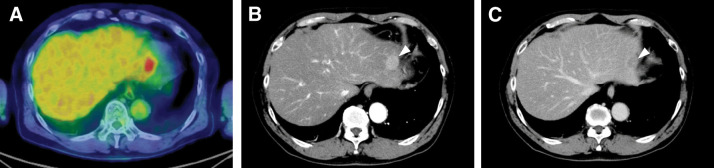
Preoperative PET-CT and dynamic CT findings of the primary hepatic lesion. (A) PET-CT demonstrated FDG uptake in the primary lesion (SUVmax 5.03). No definite abnormal uptake corresponding to the triangular ligament lesion was identified preoperatively. (B) Dynamic contrast-enhanced CT demonstrated an approximately 2.5-cm tumor at the peripheral portion of the left lateral section, showing arterial-phase hyperenhancement (arrowhead). (C) On the delayed/equilibrium phase, the same lesion showed washout (arrowhead), consistent with hepatocellular carcinoma. FDG, fluorodeoxyglucose; SUVmax, maximum standardized uptake value
